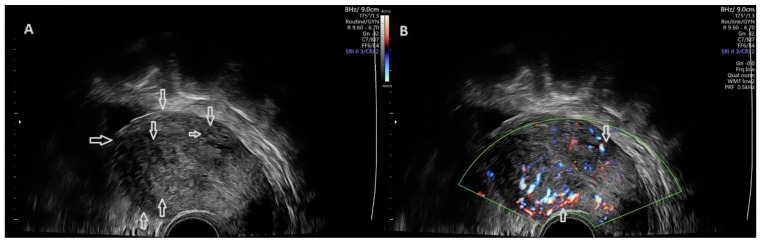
(A) B–mode, TVUS characteristics of uterine adenomyosis (featured in the superior part of the image) and a uterine fibroid (inferior part of the image) (pointed out through the arrows): globally increased uterine volume with myometrial cysts and ill-defined lesion; round lesion, with clear, distinct borders and fan-shaped shadowing. (B) B–mode TVUS with Power Doppler mode features of uterine adenomyosis (superior part of the image) and uterine fibroid (inferior part of the image) (highlighted by the arrows): trans–lesional blood flow; circumferential blood flow.
